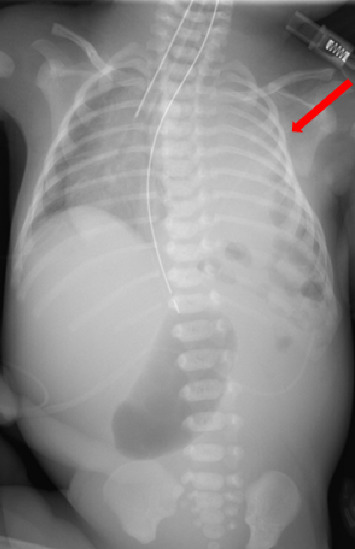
Chest X-ray image taken when entering PICU. The intestinal tract has prolapsed into the thoracic cavity, and the permeability of the left lung field has decreased.
